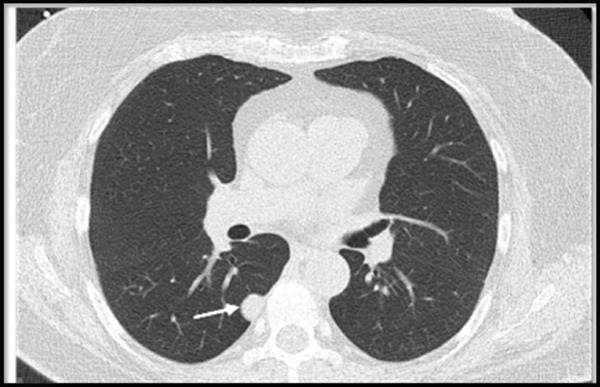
CT scan demonstrating a well‐demarcated homogenous noncalcified nodule measuring 1.8 × 1.6 cm.
